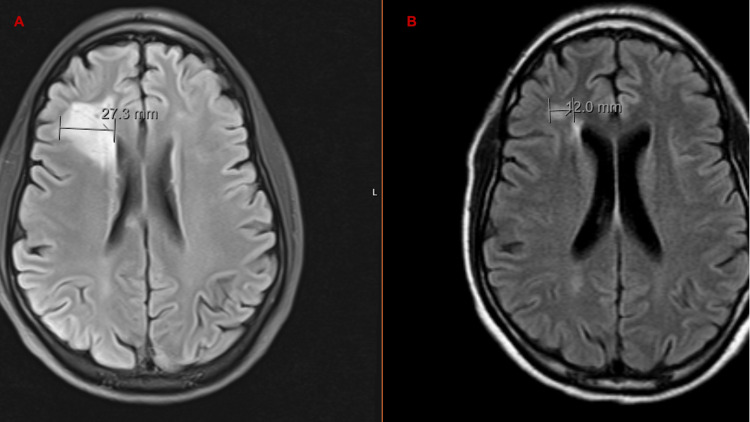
(A) MRI of the brain with enhancing lesion in the right frontal lobe. (B) MRI of the brain with resolving lesion.
